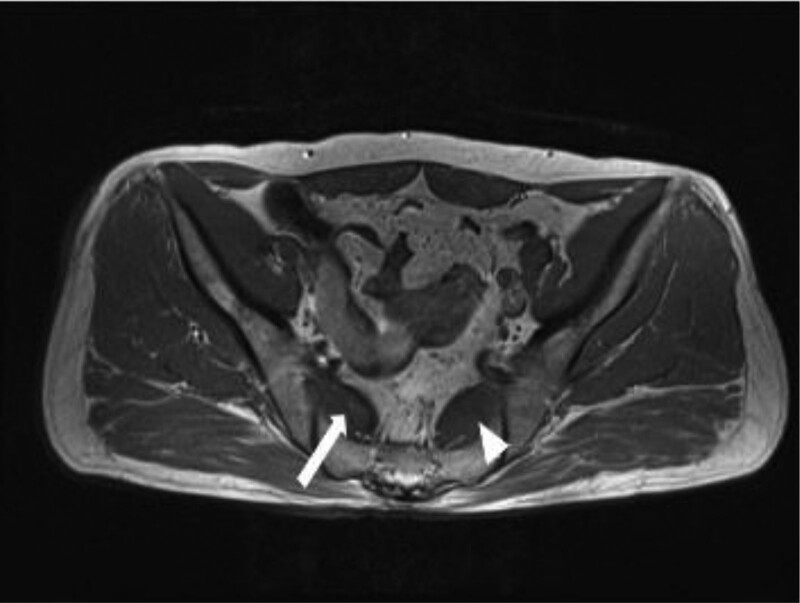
MRI axial T1-weighted image showing asymmetry of the piriformis muscles, with hypertrophy of the left piriformis muscle (white arrow) than right (white arrowhead).
